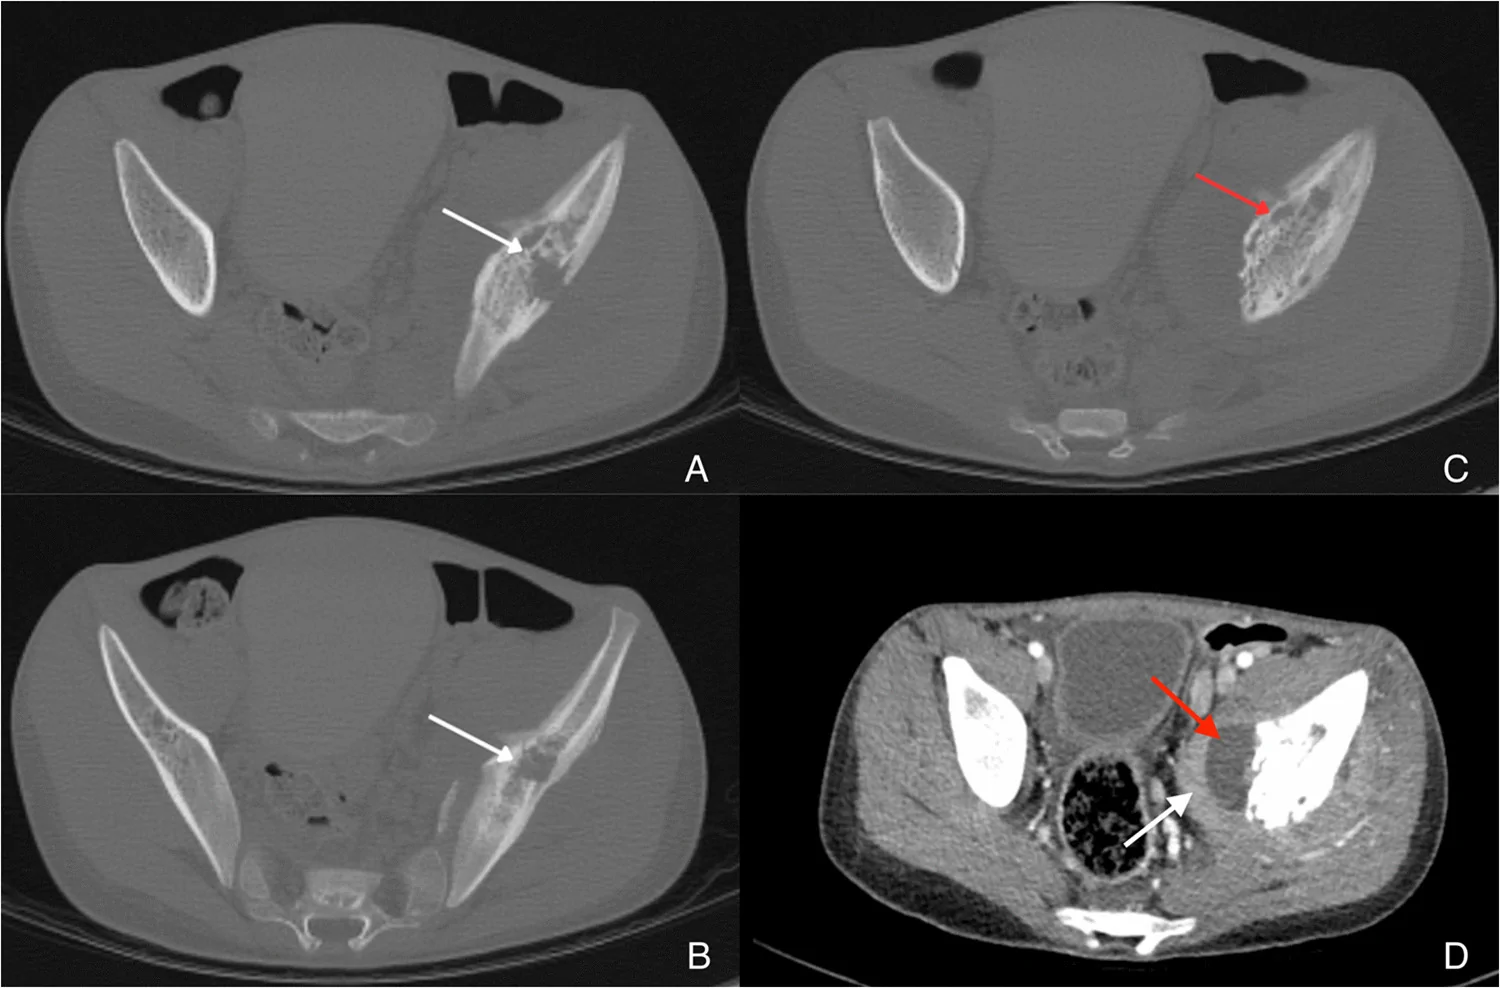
Axial computed tomography bone windows revealed cortical destruction (white arrows) (A, B) and the cloaking periosteal reaction (red arrow) (C) of the lesion. Soft tissue window visualized a hypodense fluid collection (red arrow) with obscuration of muscle planes representing edema (white arrow) (D)
